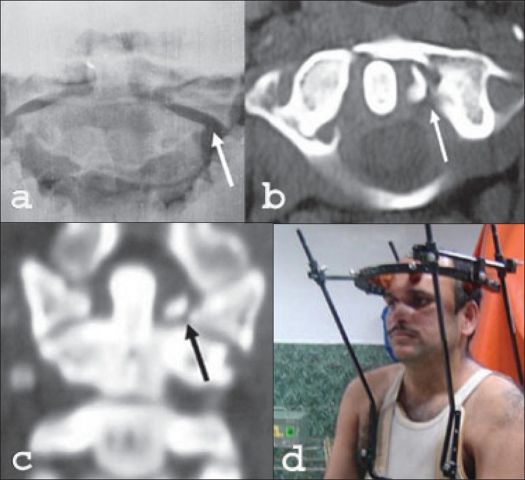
32 years old male with Jefferson fracture. Open mouth x-ray (a) shows lateral subluxation of C1-C2 joint (white arrow). Axial CT scan (b)on the left side (white arrow) and coronal reconstruction (c) (black arrow) shows the lateral subluxation. (d) The patient was treated with halo vest immobilization
